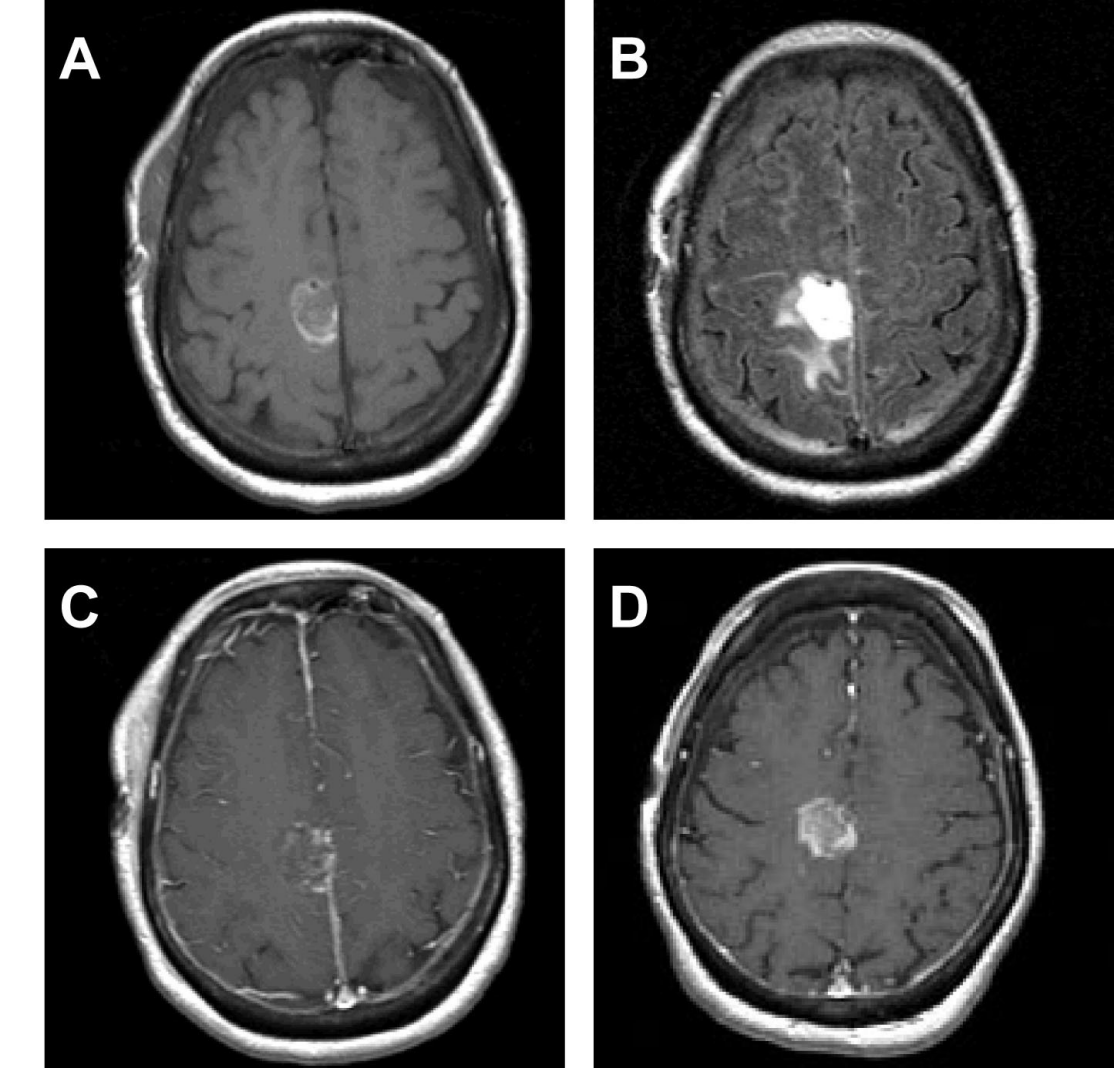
Case Example 1 This 62-year-old female underwent gross total resection of a right parietal small-cell lung carcinoma metastasis with planned adjuvant gamma knife radiosurgery. Her initial postoperative T1 weighted MRI (A) showed a resection cavity volume of 7.2 cm^3^ with 11.72 cm^3^ of surrounding edema on T2 weighted imaging (B). No enhancement was seen within the initial resection cavity (C). Her pre-SRS MRI was performed 19 days later and revealed a resection cavity of 5.9 cm^3^ (D), resulting in a volume reduction of 18%. The resection cavity also had evidence of local tumor progression on contrast-enhanced imaging (D).
